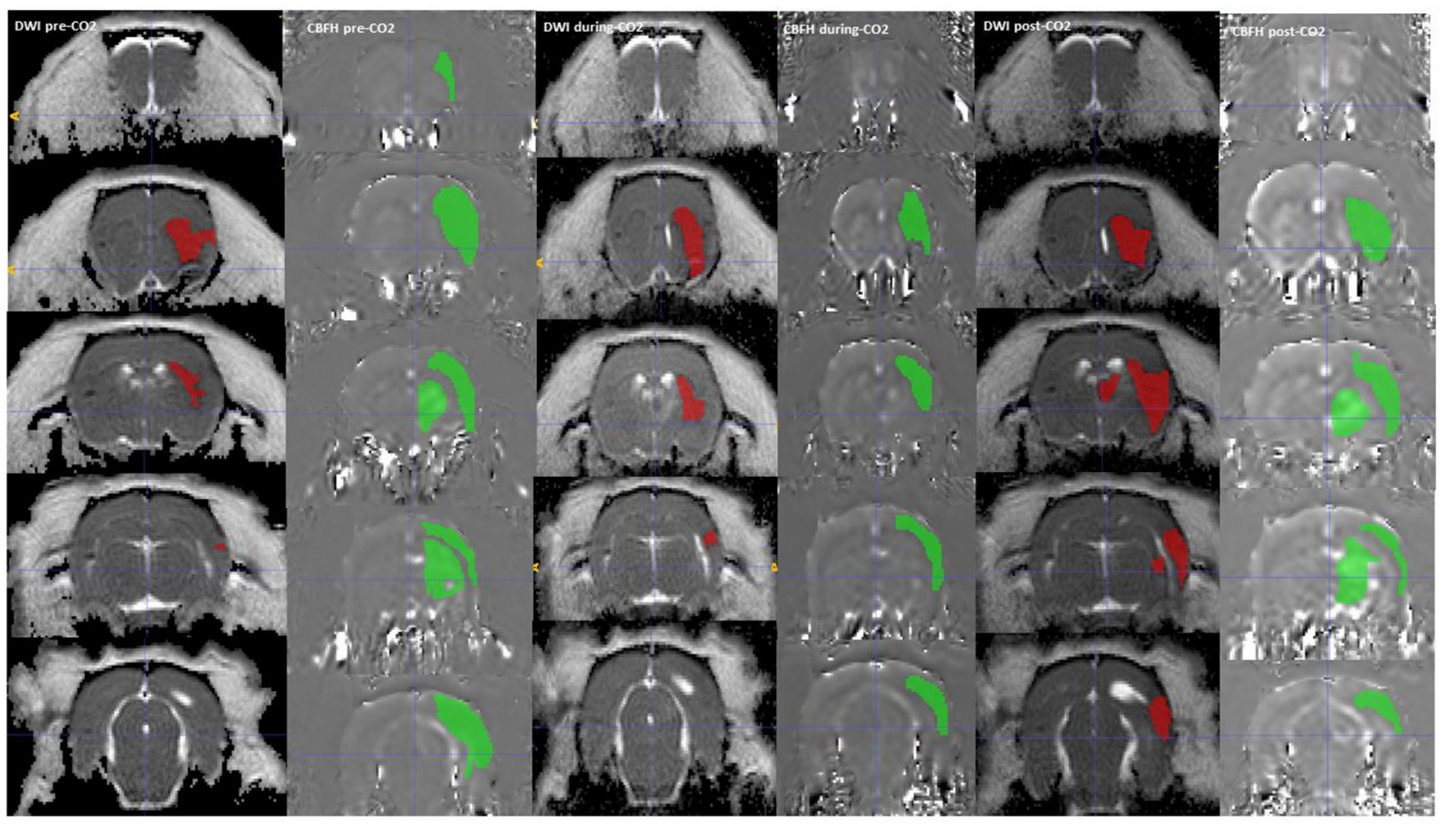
A DWI (Green) and CBF hypoperfusion, CBFH (Red) region of interest drawn onto the corresponding MRI sequences, showing a reduction in CBF hypoperfusion volume with administration of CO_2_. The CBF hypoperfusion volume reverses and increases with stoppage of CO_2_.
